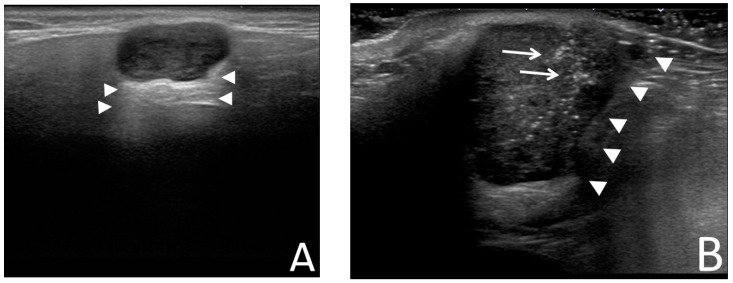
The subjective ultrasound features of SGTs. Note: (A) Benign SGTs are usually characterized by well-defined, homogeneous, and solid masses with posterior enhancement (arrowheads). (B) On the other hand, malignant SGTs tend to exhibit indistinct boundaries, irregular shapes (arrowheads), the presence of calcifications (arrows), and a lack of posterior enhancement. Abbreviation: SGT: salivary gland tumor.
